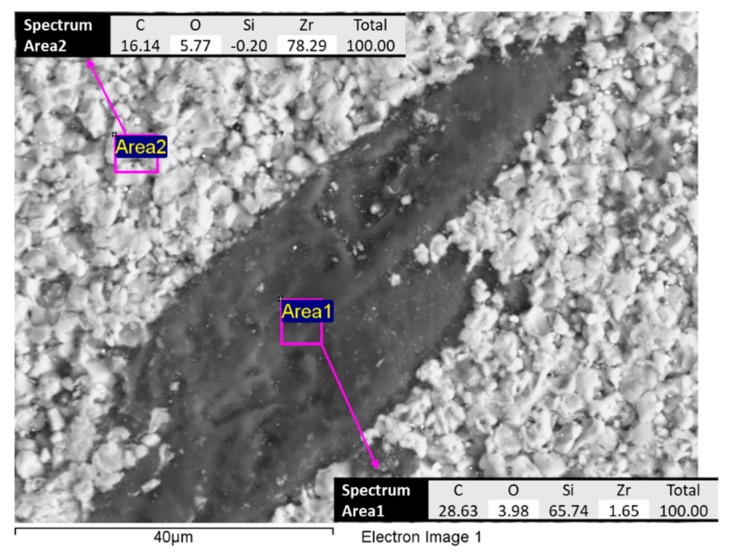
Example of an energy dispersive X-ray (EDX) analysis of the base material ZrB5.
